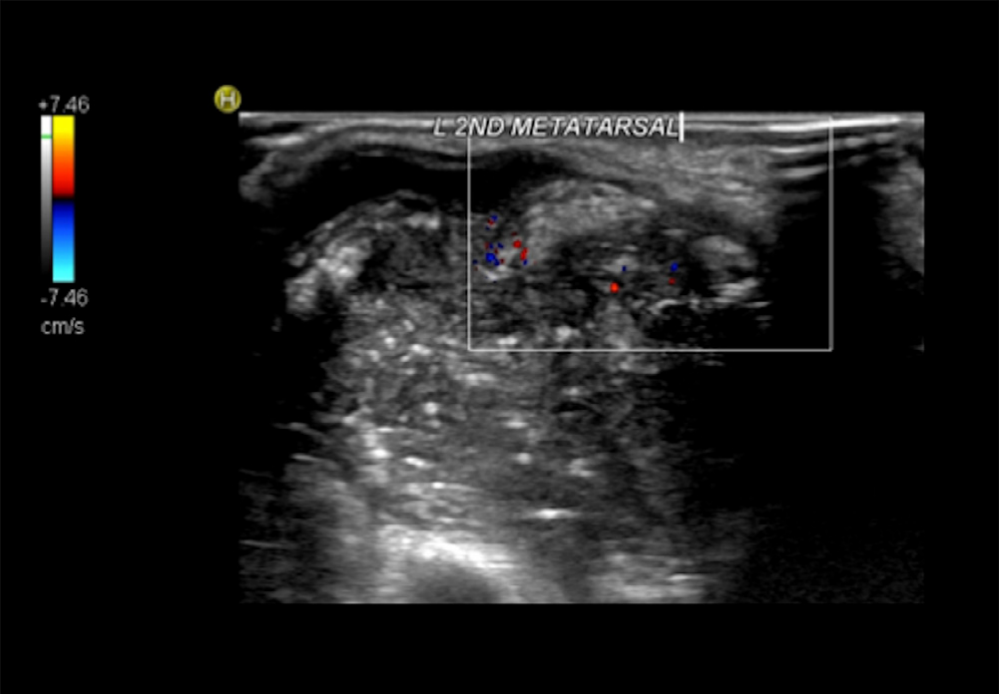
Transverse color Doppler ultrasound of the left foot focused on the soft tissue mass at the neck of the second metatarsal demonstrating increased vascularity at the periphery of the lesion.
